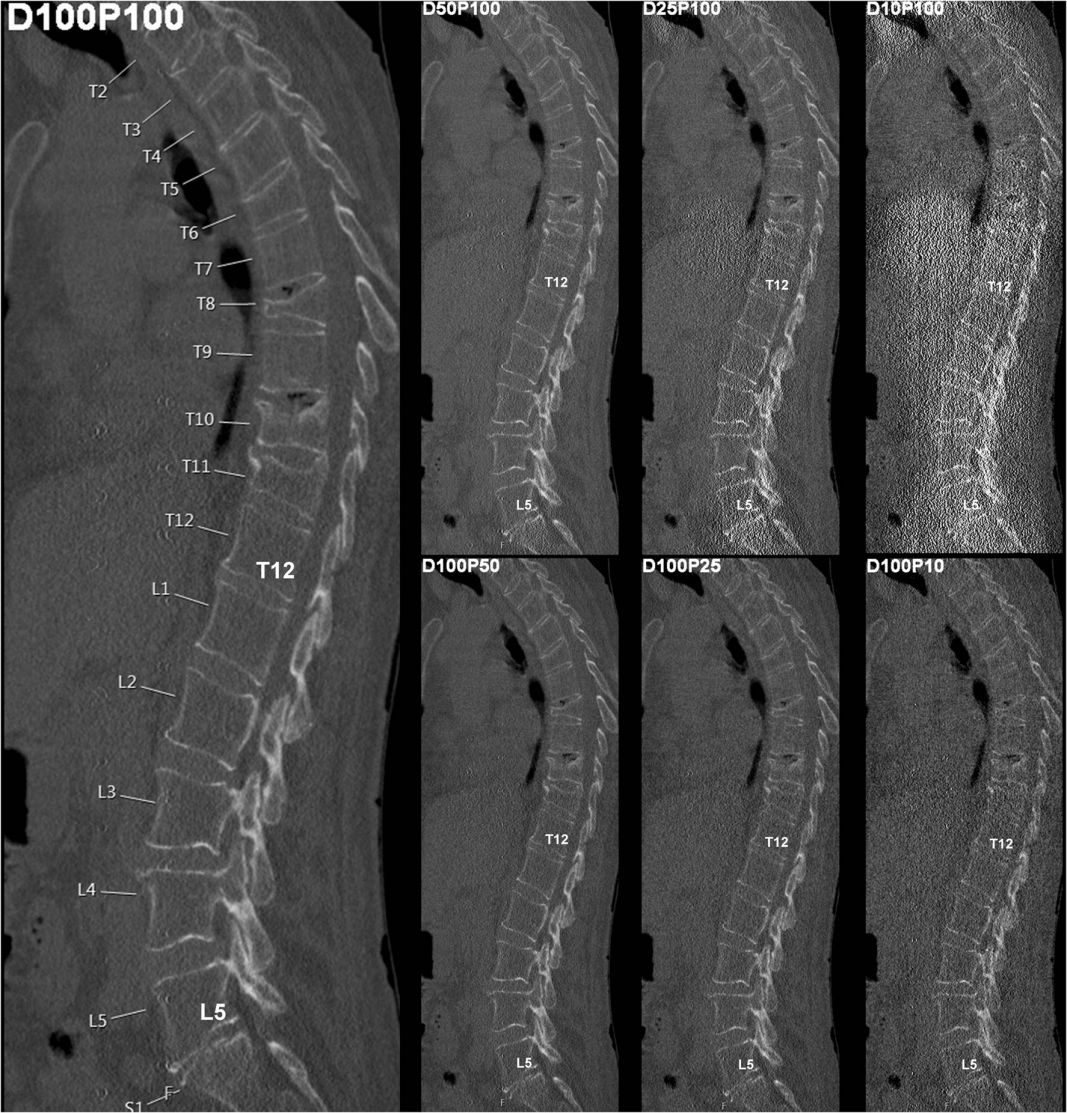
Virtual tube current reduction and sparse sampling in multi-detector CT (MDCT) of the thoracic and lumbar spine. Sagittal slices derived from full-dose MDCT (D100 P100), MDCT with virtually lowered tube current (D50 P100, D25 P100, and D10 P100), and MDCT with sparse sampling (D100 P50, D100 P25, and D100 P10) are shown in a patient with five thoracic fractures (T3, T6, T8, T10, and T11)
